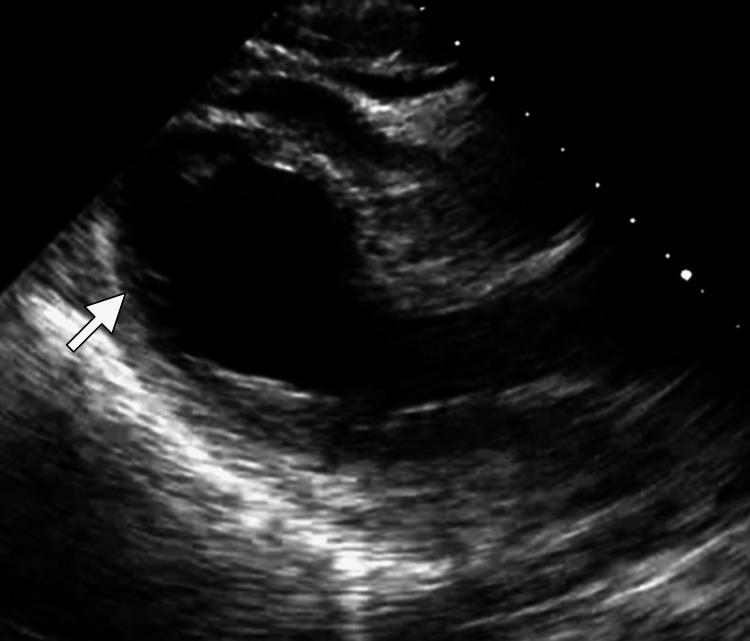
Three-chamber echocardiogram demonstrating hypokinesis of the mid to apical segments of the left ventricle (arrow) The apical and mid-ventricular regions showed reduced movement, characteristic of takotsubo cardiomyopathy, with preserved basal segment contractility. This echocardiographic pattern, often described as "apical ballooning," highlights the transient regional wall motion abnormalities associated with the condition.
